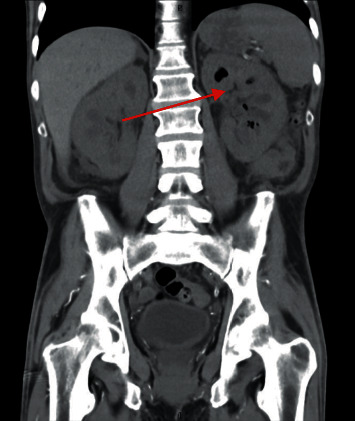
Coronal CT image showing evidence of emphysematous pyelonephritis (red arrow).
